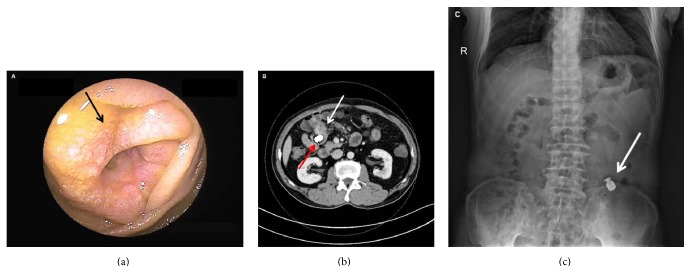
Images in a patient presenting with melena and diagnosed with adenocarcinoma. Capsule endoscopy was retained near the lesion, with no clear picture showing the lesion. (a) Image from double-balloon enteroscopy indicated a circumferential protruding lesion (arrow) with narrowed lumen in the proximal jejunum. (b) Dual-phase computed tomographic enterography showed a protruding lesion (white arrow) and retained capsule (red arrow) in the jejunum. (c) X-ray revealed the retained capsule (arrow).
